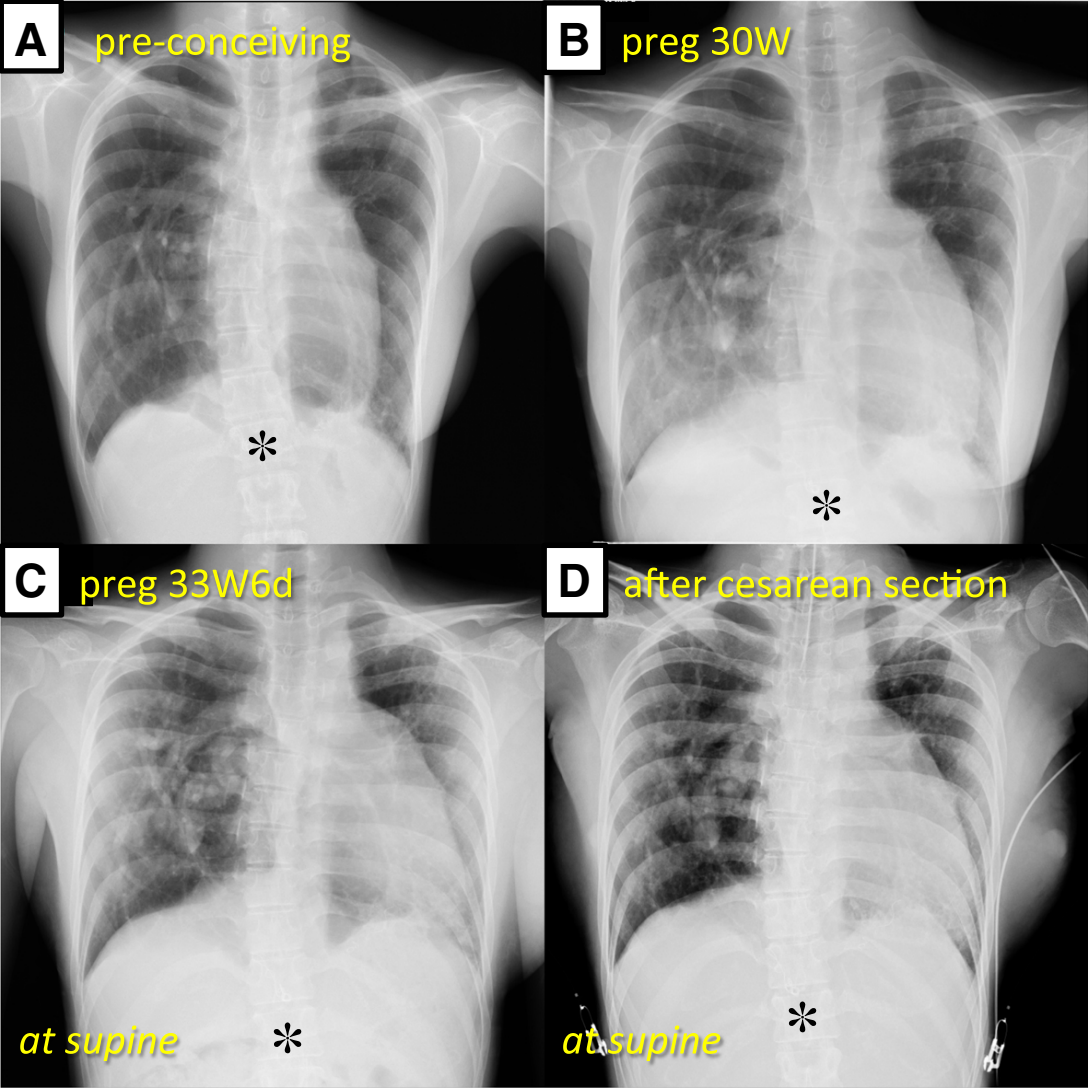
a Chest X-ray, at pre-conception, demonstrated that the right lung looked over-infiltrated and was bulging into the left thorax. b At 30 weeks of gestation, the position of the diaphragm was located at the same level as before conception, while vascular shadows in the right lung field were increased. (Asterisk marks the 12th thoracic vertebra in each chest X-ray.) c On the day before cesarean section, chest X-ray in supine position confirmed no significant displacement of the diaphragm, when considering the impact of the posture on diaphragm position; however, it revealed further increase of vascular shadows in the right lung field. d No abnormal findings in the lung field and no significant change of the diaphragm level, compared with those the day before the operation, were confirmed, immediately after the cesarean section
